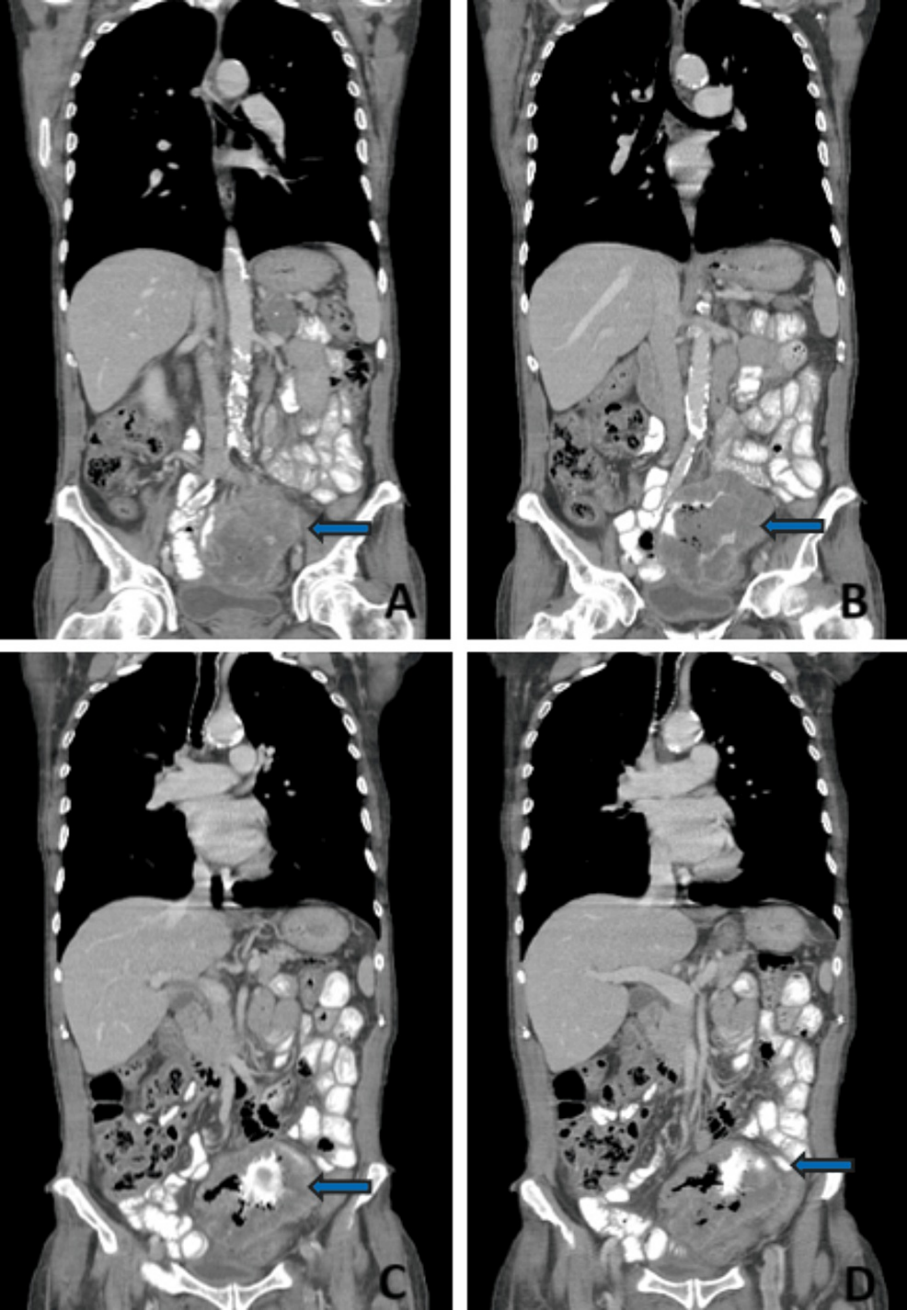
Thoracoabdominopelvic CT scan, coronal cuts A, B, C, and D: posterior to anterior coronal cuts; blue arrows: abdominal mass with an ileocolic fistula
